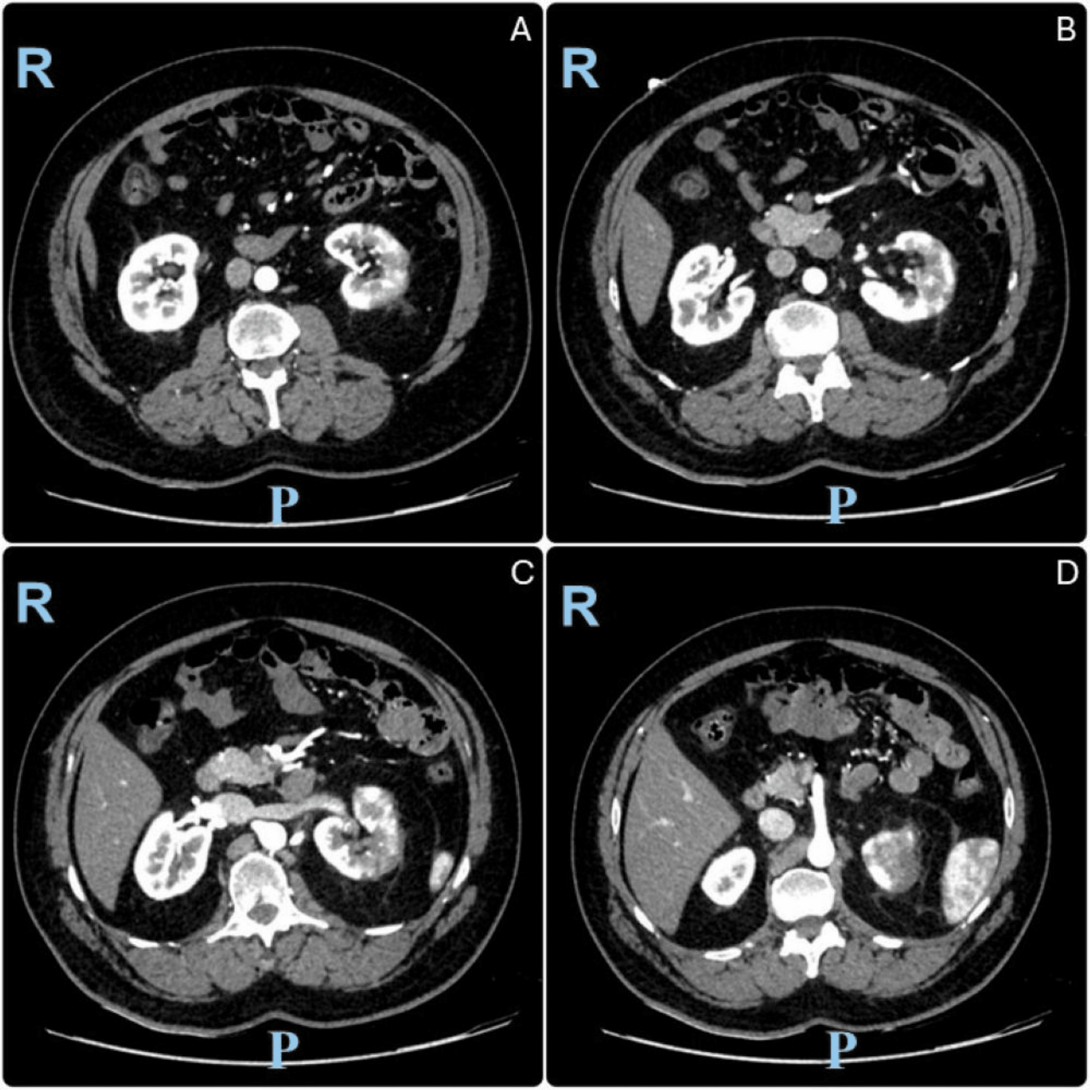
Axial view computed tomography of the abdomen and pelvis with intravenous contrast in the arterial phase showing multifocal left renal infarcts Images are ordered in a caudocephalic direction. R: right side; P: posterior side
